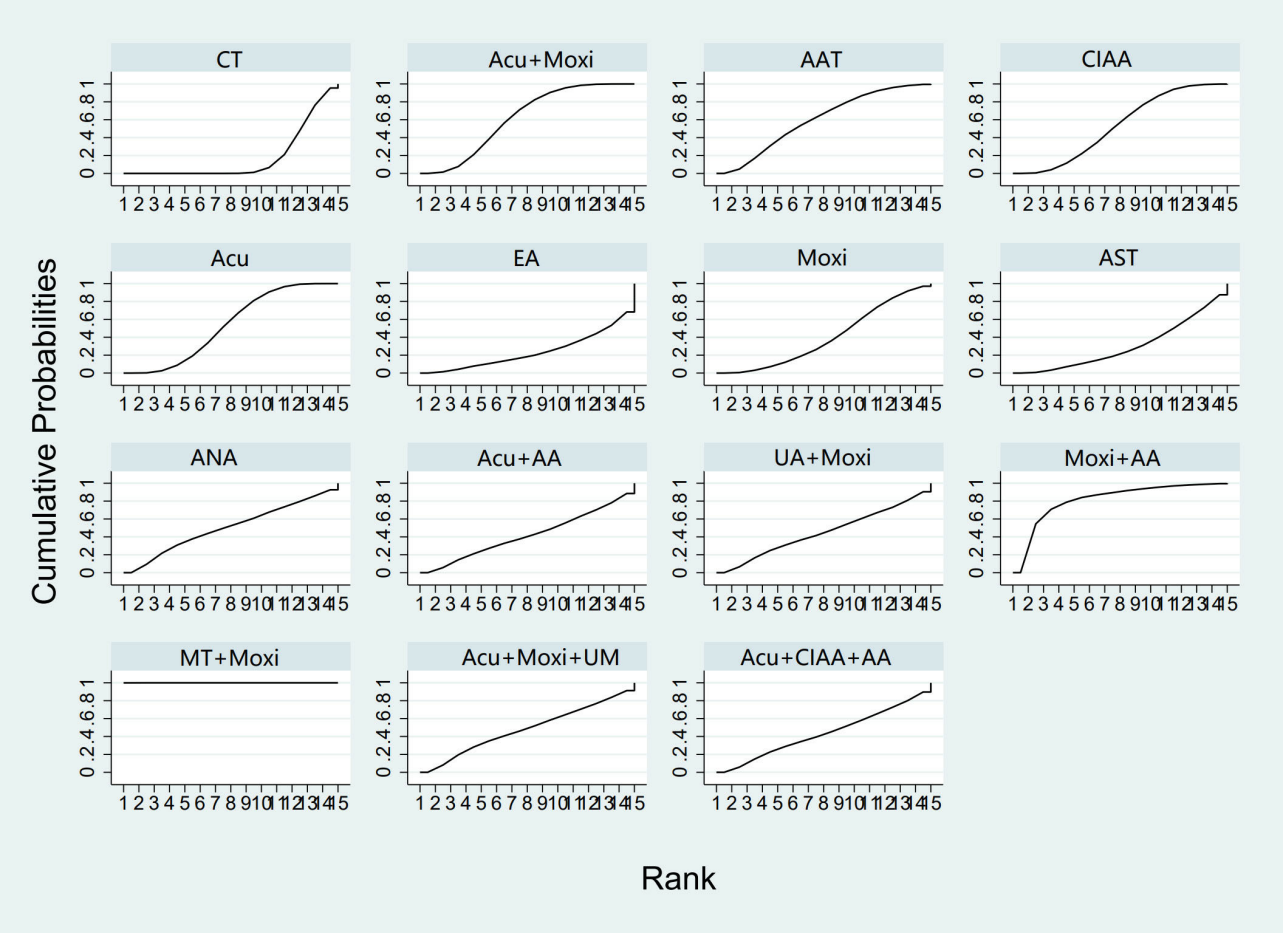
Efficacy ranking and cumulative probability graph of estradiol (E_2_) treated by different types of intervention. CT, conventional therapy; Acu, acupuncture; Moxi, moxibustion; AAT, abdominal acupuncture therapy; CIAA, catgut implantation at acupoint; EA, electroacupuncture; AST, auricular seed therapy; ANA, awn needle acupuncture; UA, umbilical acupuncture; AA, acupoint application; MT, massage therapy; UM, umbilical moxibustion.
